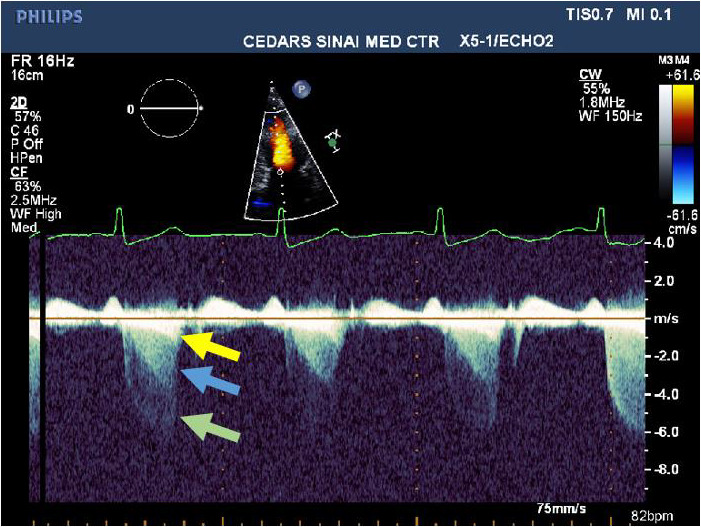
Spectral continuous‐wave Doppler profile in a patient (not in the study) with overlapping (in order of peak velocity) mitral regurgitation (green arrow), SAM‐associated LVOT obstruction (blue arrow), and LVCO (yellow arrow), demonstrating depth ambiguity
